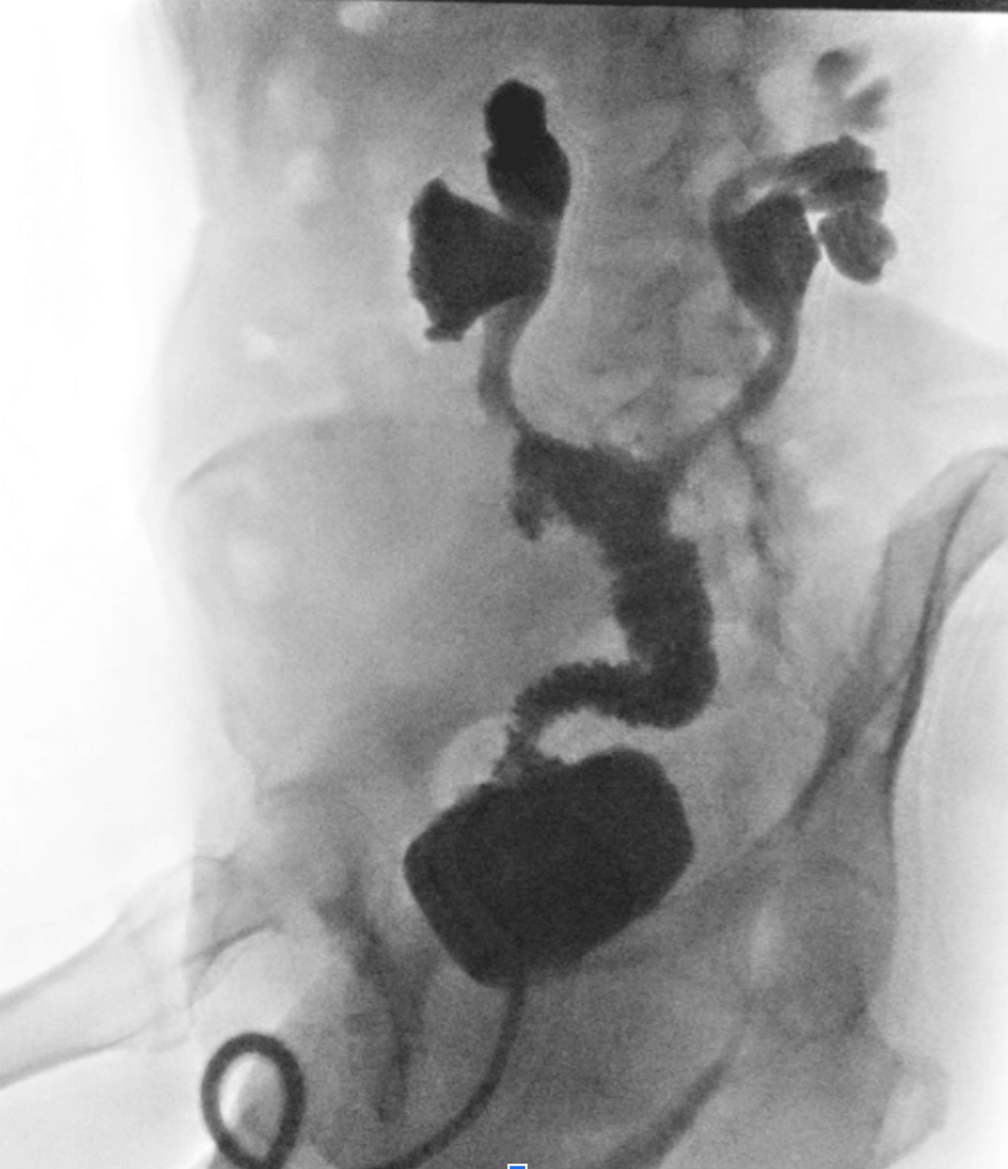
Post-procedural X-ray cystogram Demonstrating no evidence of leak/obstruction status post distal ureterectomy with interposed ileo-bladder anastomosis. Free contrast reflux to the kidneys with bilateral hydronephrosis.
